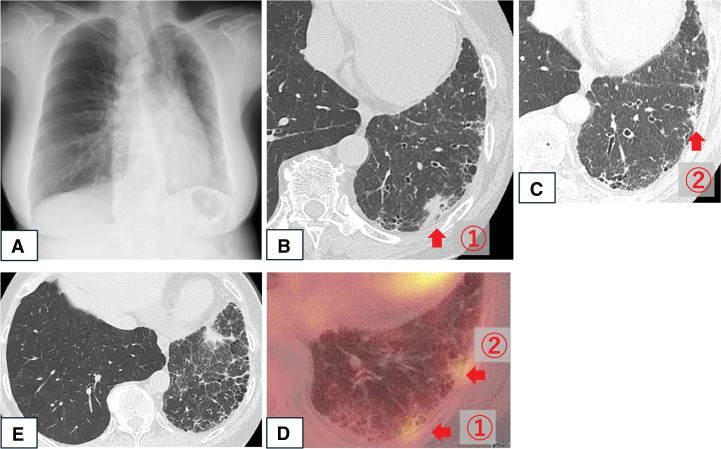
(A) Chest radiography showed left lung volume loss, leftward mediastinal shift, and reticular shadows. (B–D) CT showed ① a 25-mm solid nodule in left S9 with FDG uptake (SUVmax 4.02), ② a 10-mm nodule in left S8 with FDG uptake (SUVmax 3.03). (E) Unilateral interstitial pneumonia in the left lung. FDG, 18F-fluorodeoxyglucose; S8, segment 8; S9, segment 9
